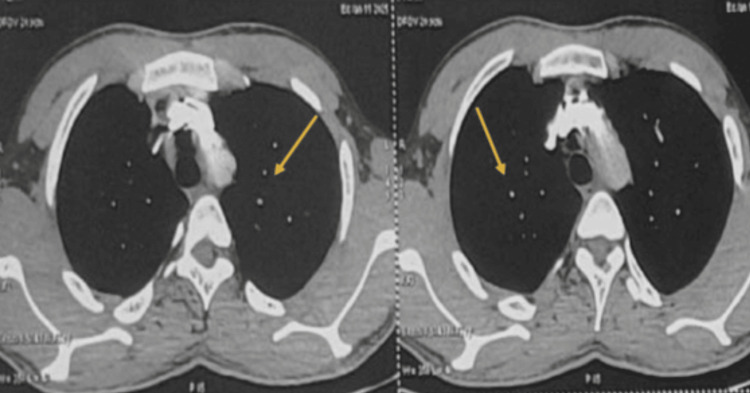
Axial contrast-enhanced CT of the thorax (mediastinal window) from CT pulmonary angiogram (CTPA) showing centrilobular nodules with subpleural and fissural sparing in both lungs (arrows), suggestive of talc granulomatosis. CT: computed tomography
